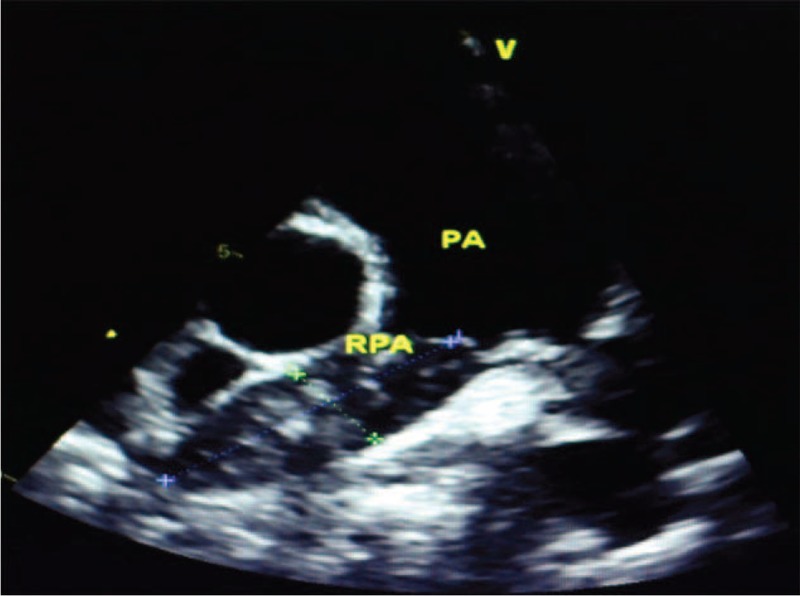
Echocardiography revealed the right ventricule was occupied by a dense sessile mass.
